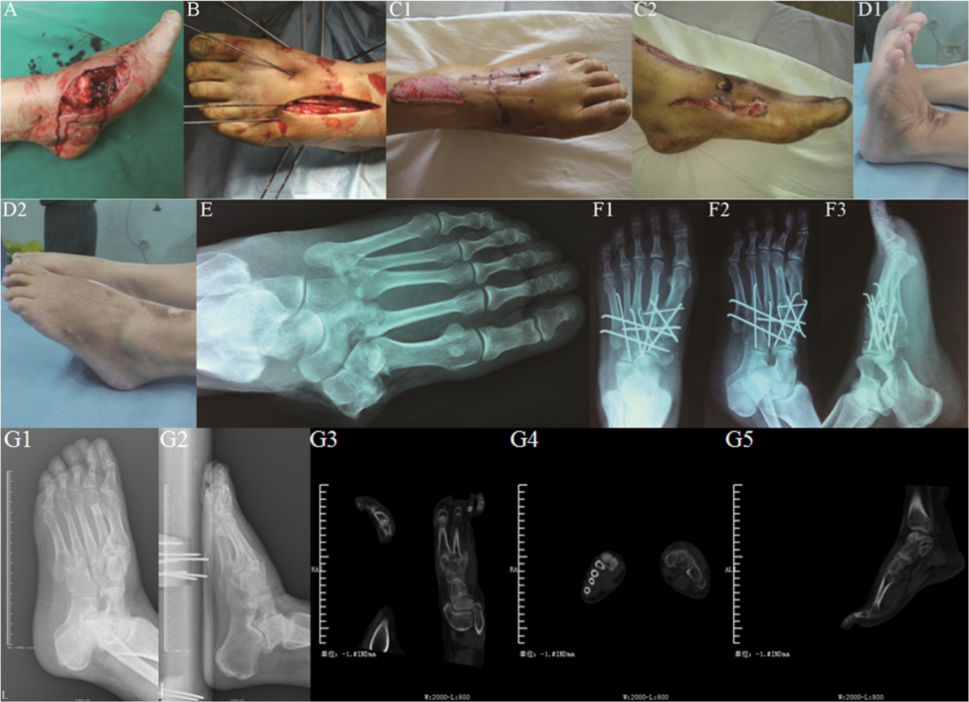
Female patient, aged 52, with left foot severe open Lisfranc fracture-dislocation, was transferred to our hospital 3 h after traffic injury. One-stage internal fixation using k-wires associated with VSD was performed during emergency. a Severe open wound on the left foot with bone exposure. b One-stage reduction and multiple k-wire internal fixation were performed via the open wound and dorsal incision, sealed with VSD kit. c The condition of original wound and incision was checked when VSD kit was changed 4 days after surgery. d Four months after surgery when the internal fixators were removed, with satisfying appearance and function. e On X-ray film before surgery, fracture-dislocation was observed at the left Lisfranc joint. f AP, oblique, and lateral X-ray showed that the fracture-dislocation reached to anatomical reduction after one-stage internal fixation. g X-ray film and CT scan showed good matching midfoot joint after removing the internal fixation at half a year operation
